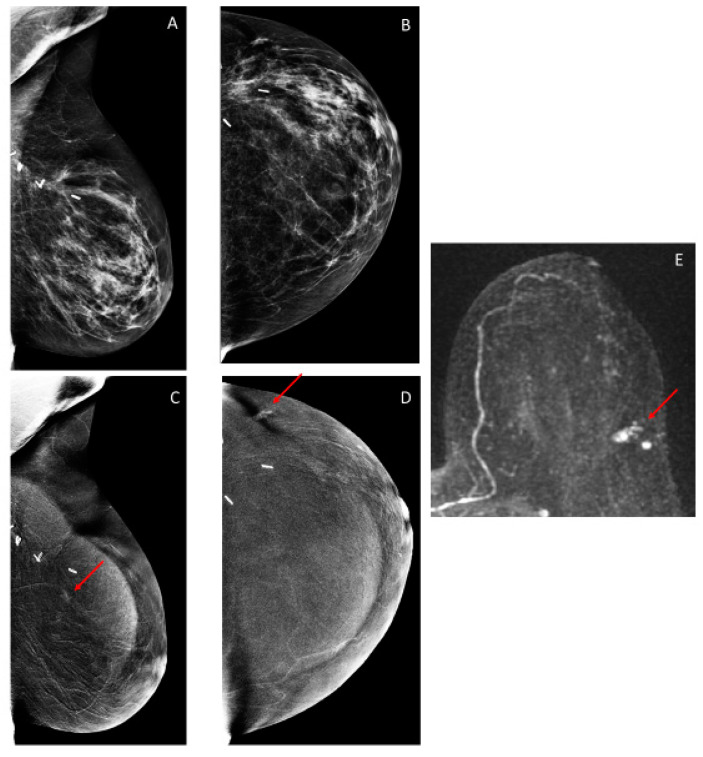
Sixty-six-year-old woman treated with left breast conservation 3 years prior for invasive ductal carcinoma (IDC) and ductal carcinoma in situ (DCIS). (A,B) Low-energy images show postsurgical distortion but no suspicious findings. (C,D) Iodine images show clumped non-mass enhancement in the outer central breast (arrows). Targeted ultrasound showed no correlative finding. MRI shows non-mass enhancement spanning 1.3 cm ((E) left breast subtraction maximum intensity projection (MIP)); the enhancement was biopsied with MRI guidance, yielding IDC and DCIS. The patient was then treated with a mastectomy.
